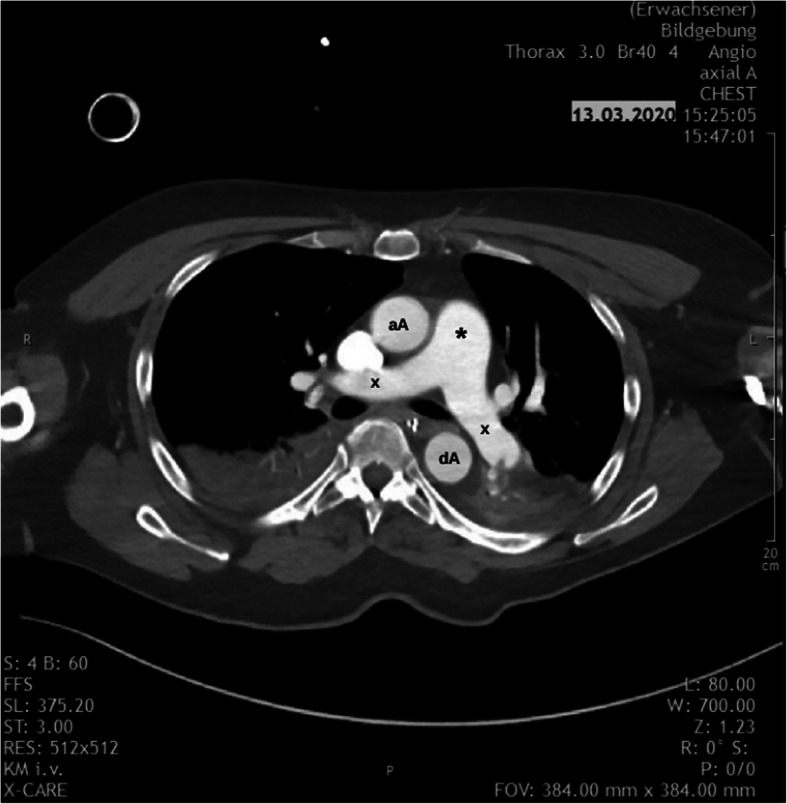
CT scan revealed regredient embolic burden (x) and improved recanalization after ECMO explantation (* = pulmonary trunk, aA = ascending aorta, dA = descending aorta)
